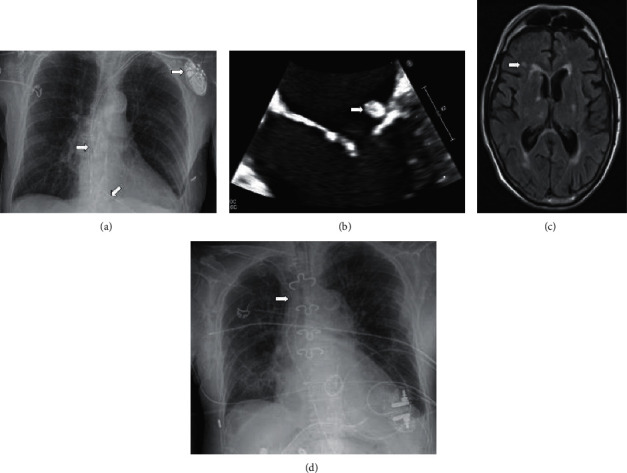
(a) Chest radiography with implanted pacemaker, (b) transesophagal echography, (c) MRI cerebral showed the emboli, and (d) chest radiography with external pacemaker.
